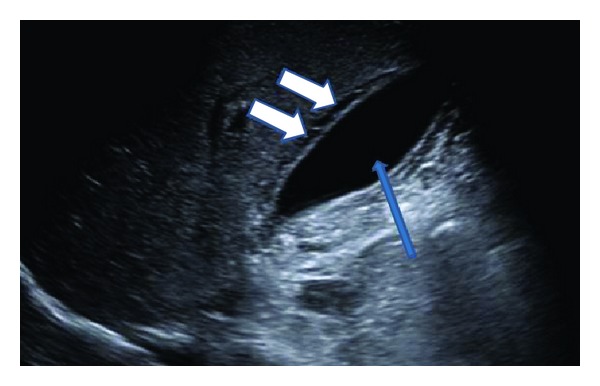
Ultrasound of the right upper quadrant showing the gallbladder free of stones (blue arrow) and thickening of gallbladder wall (white arrows).
